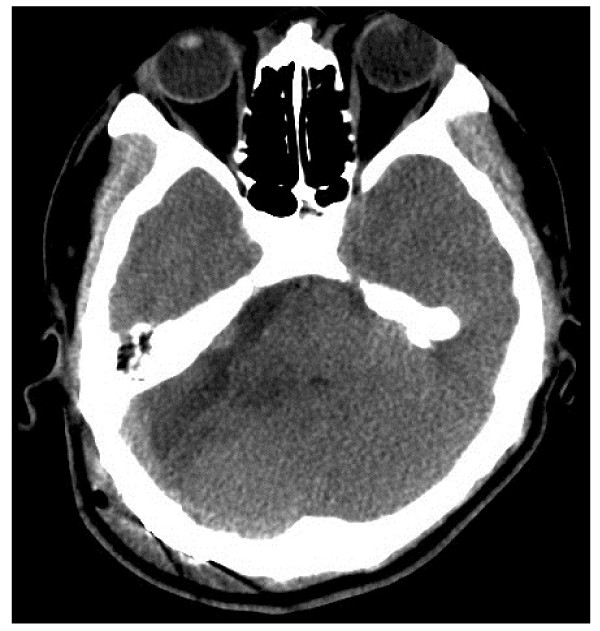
Postoperative CT examination showed that the CMs had been completely removed.
